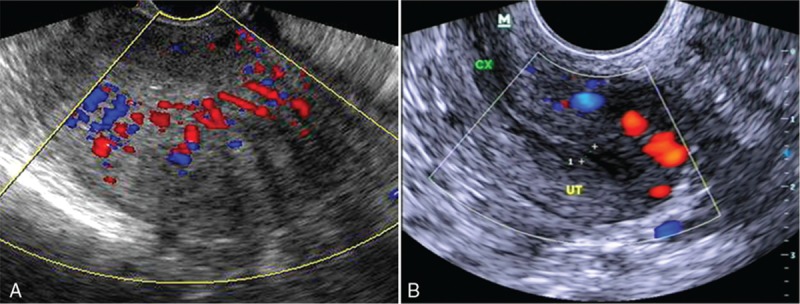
The patients with adenomyosis took B ultrasonic examination before or after the operation. Before the operation, we found enlarged uterus with rich blood flow signal. After the operation, the uterus was shrunk. (A) Before the operation, the uterus was enlarged with rich blood flow signals. (B) After the operation, the uterus was shrunk with poor blood flow signals.
